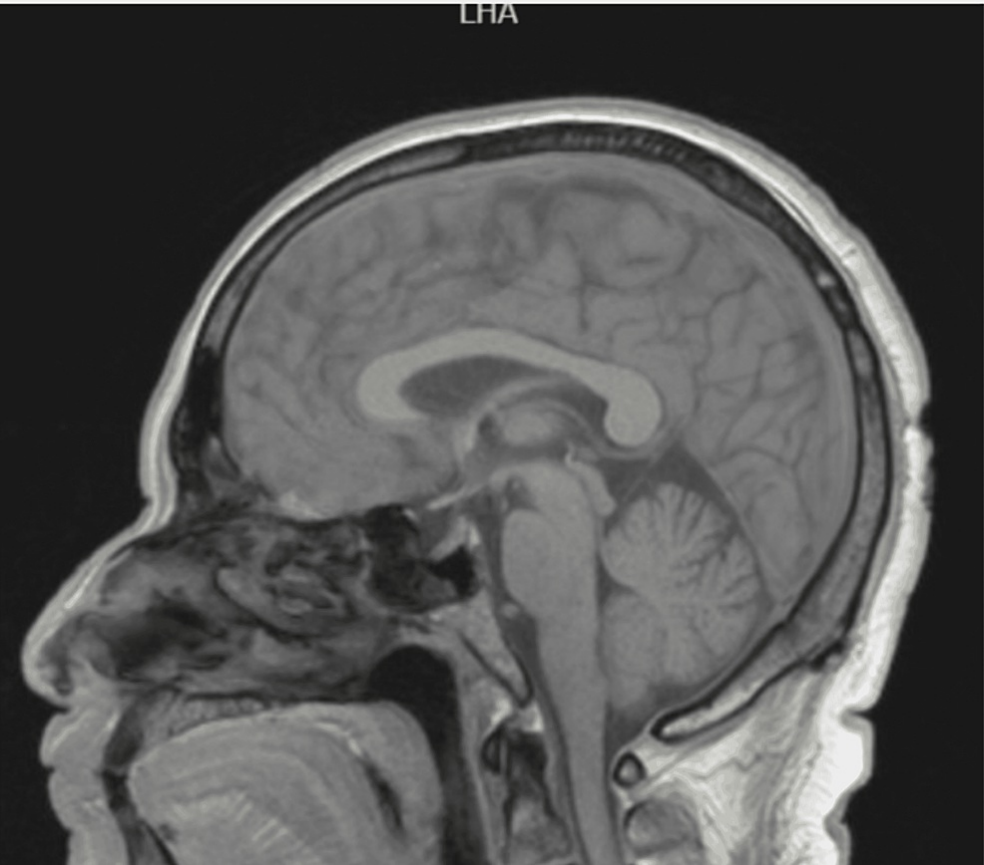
MRI Sagittal T1. MRI brain showing a cerebral arachnoidocele.
